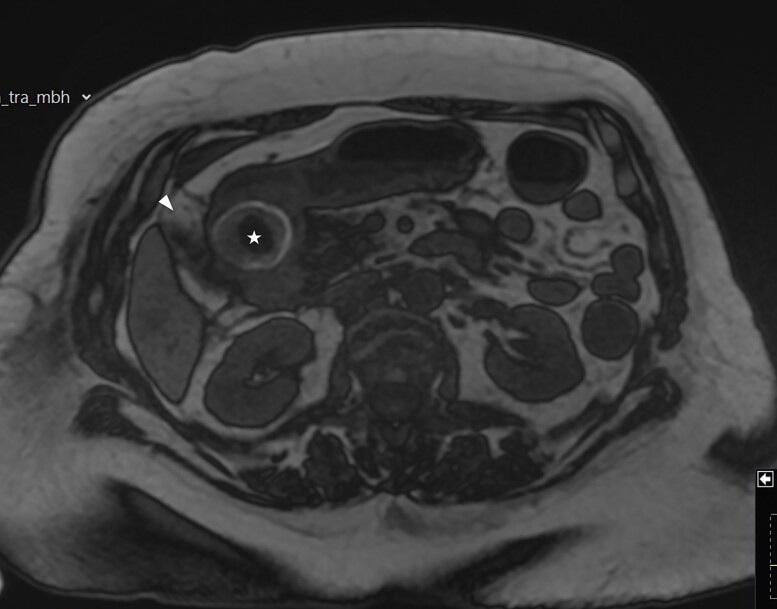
MRI scan showing the gallbladder adherent to the duodenum with an impacted stone in the duodenum.
